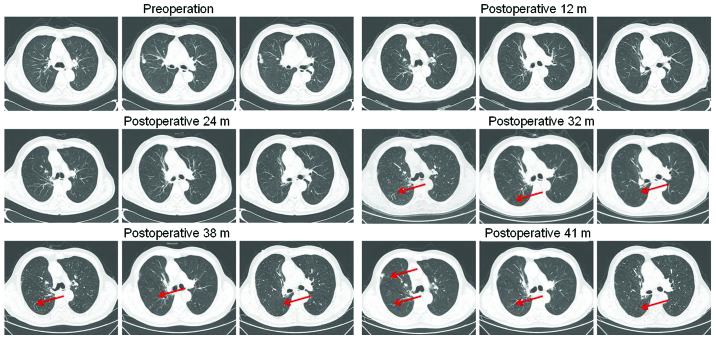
Computed tomography (CT) scans of the patient. No evident changes were observed in the chest radiographs until 32 months following surgery, when multiple patchy shadows were observed on the right lung. After a further nine months, chest CT scans revealed a subpleural nodule (0.5×0.5 cm) and a slight enlargement of the patchy shadow. m, months.
